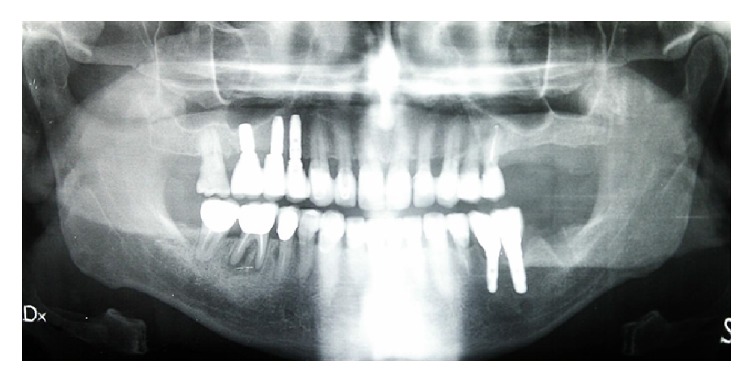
Final case, full-mouth intraoral radiographic exam.
